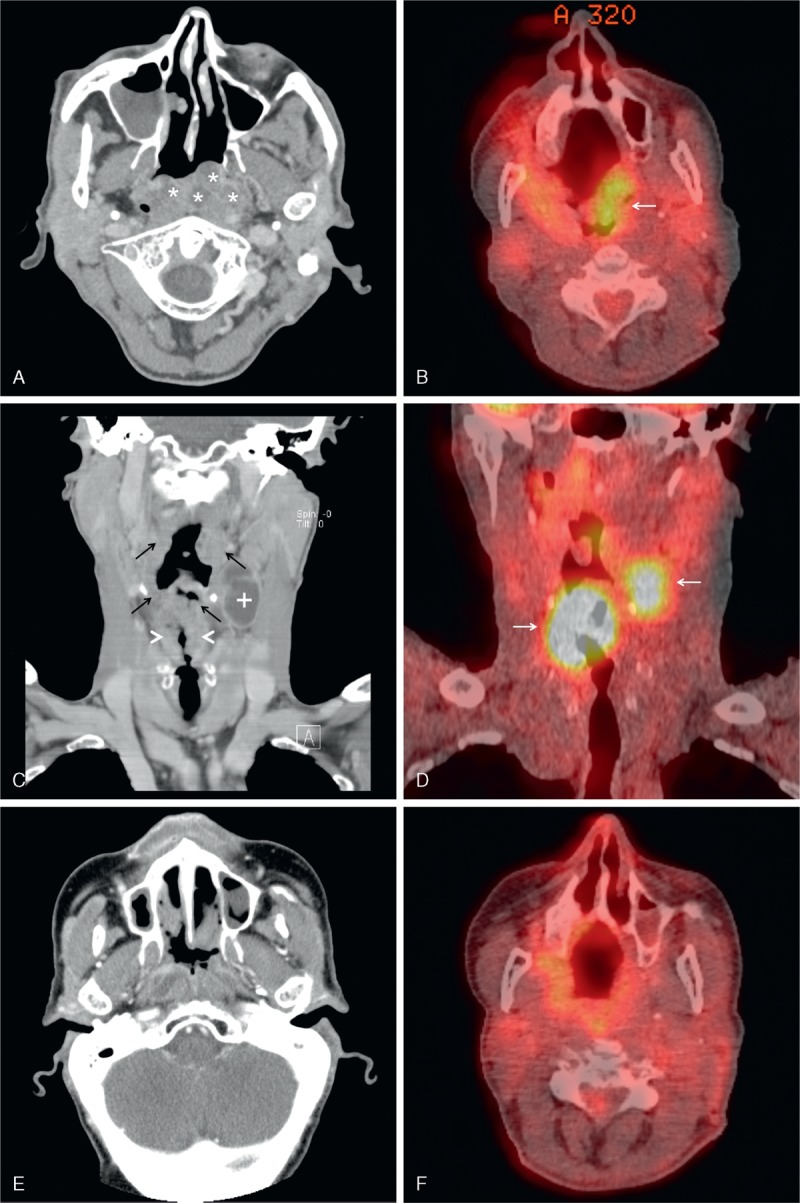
CT (A, C, E) and PET-CT (B, D, F) scans of the patient before (A–D) and after (E and F) therapy. (A) OPC with characteristic cystic lesions (asterisks) of the left epipharyngeal wall. (B) Mild FDG uptake of the OPC (white arrow). (C) OPC of the pharynx (black arrows), swelling of the supraglottic region (white arrowheads), and left-sided neck mass (plus sign). (D) High FDG uptake of supraglottic mass and neck metastasis (white arrows). (E and F) Epipharyngeal wall after therapy. CT = computed tomography, FDG = fluorodeoxyglucose, OPC = oncocytic papillary cystadenoma, PET-CT = positron emission tomography-CT.
